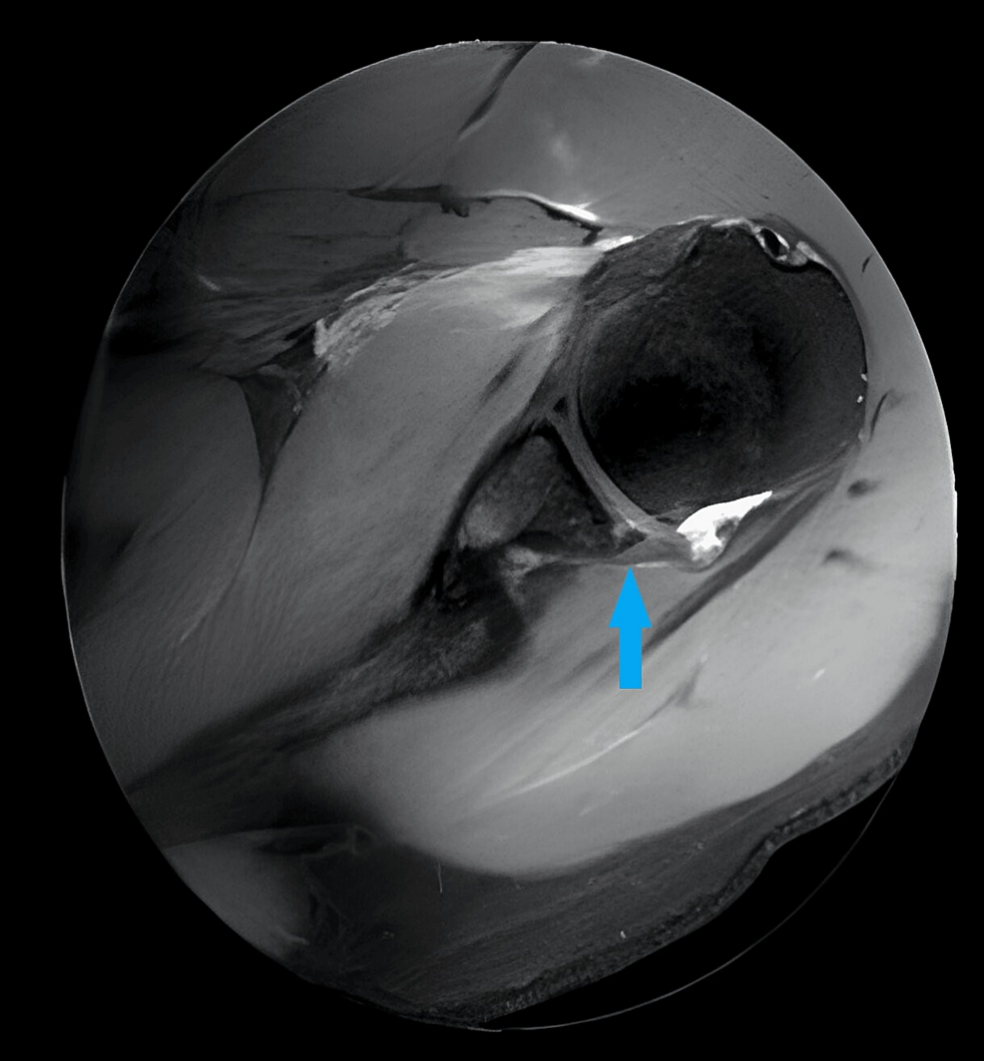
MRI scan T2-weighted axial view of right shoulder showing bony Bankart lesion (blue arrow).
